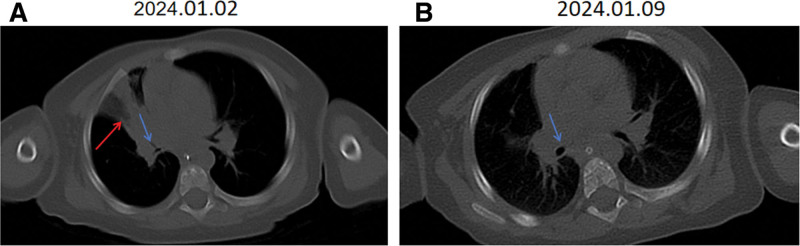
(A) The chest CT demonstrates left lung pneumonia (indicated by the red arrow) with obstructive narrowing of the left main bronchus (marked by the blue arrow). (B) One week later, a follow-up chest CT scan demonstrated complete resolution of the left lung pneumonia, with no recurrent stenosis observed in the left main bronchus (as indicated by the blue arrow). CT = computed tomography.
